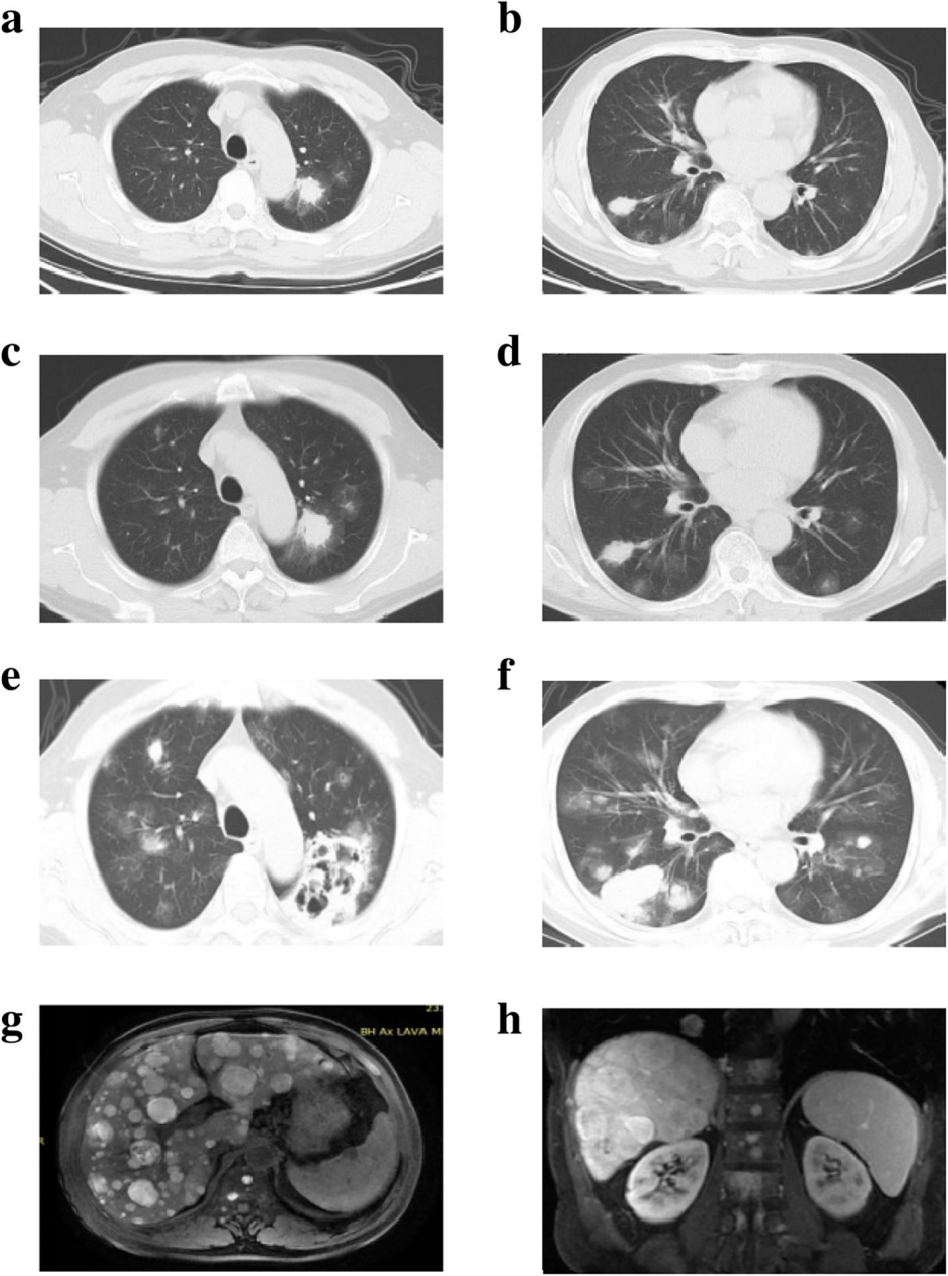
Relevant imageology characters during the patient’s visit. A-B, Chest CT on March 16th, 2020. C-D, Chest CT on June 2nd, 2020. E-F, Chest CT on Janurary 12th, 2021. G-H, Upper abdominal enhanced MRI on Janurary 10th, 2021
